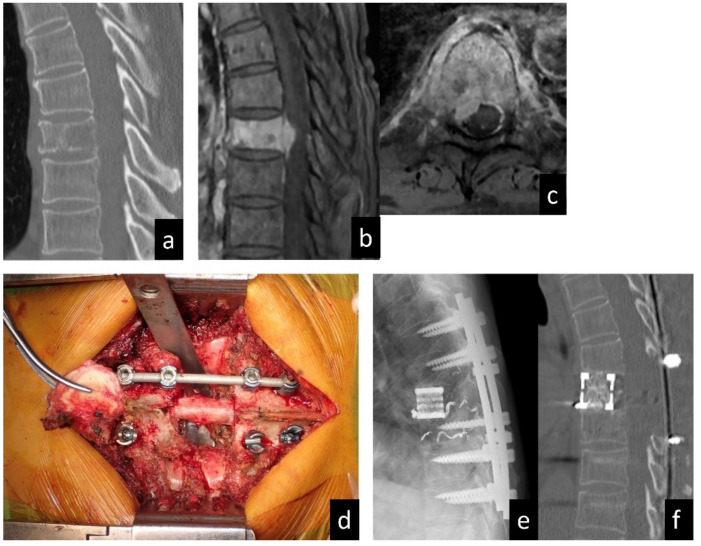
A 63-year-old man diagnosed with SM of T8 from renal cell carcinoma. (a) Sagittal plane computed tomography image showing the osteolytic lesion in the T8 vertebral body. (b) Sagittal and (c) axial images of enhanced T1-weighed magnetic resonance imaging showing spinal canal extension of the tumor. (d) Intraoperative photo in the resected vertebral body. (e) Radiograph in lateral view and (f) computed tomography in sagittal view showing the reconstructed spine after metastasectomy (total en bloc spondylectomy).
